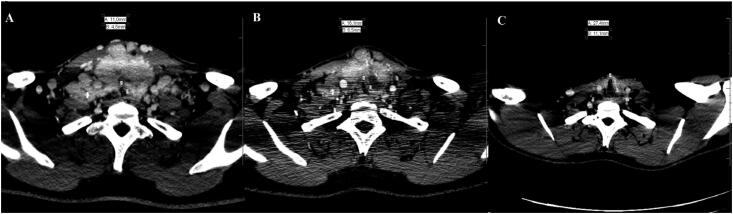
CT imaging demonstrating improvement of tracheal compression and lumen reduction. (A) Initial scan. (B) Scan after 12 months of sorafenib. (C) Last scan after 4 years of diagnosis.
